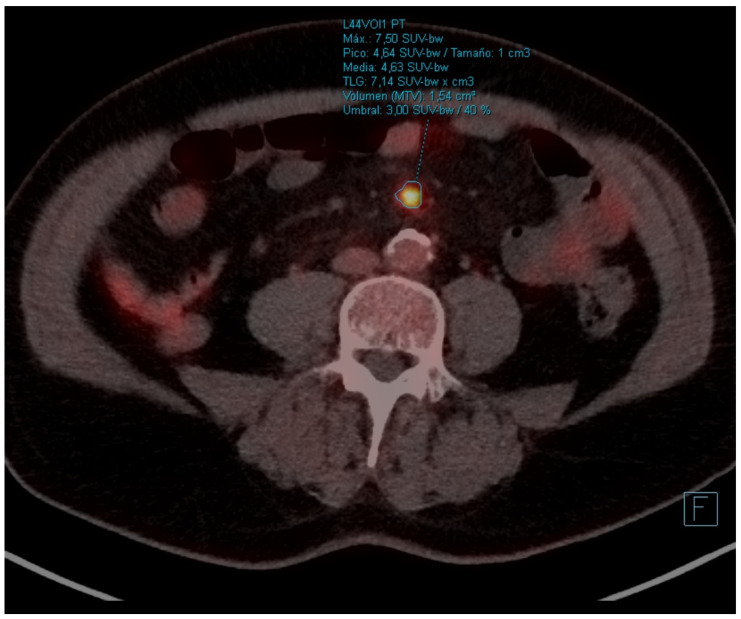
Standard PET-CT. Signs of mesenteric panniculitis, visualizing multiple pseudonodular formations several of them with FDG uptake up to SUVmax 7.50.
